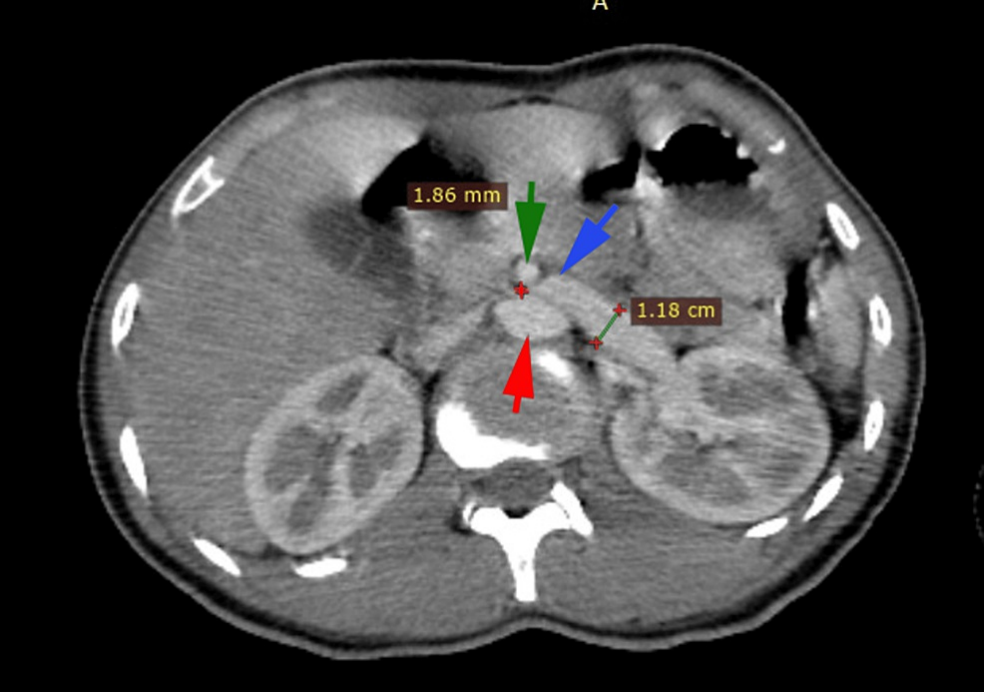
Abdominal CT scan image Tomography image demonstrating the compression of the left renal vein (blue arrow) by the aorta (red arrow) and the superior mesenteric artery (green arrow).
